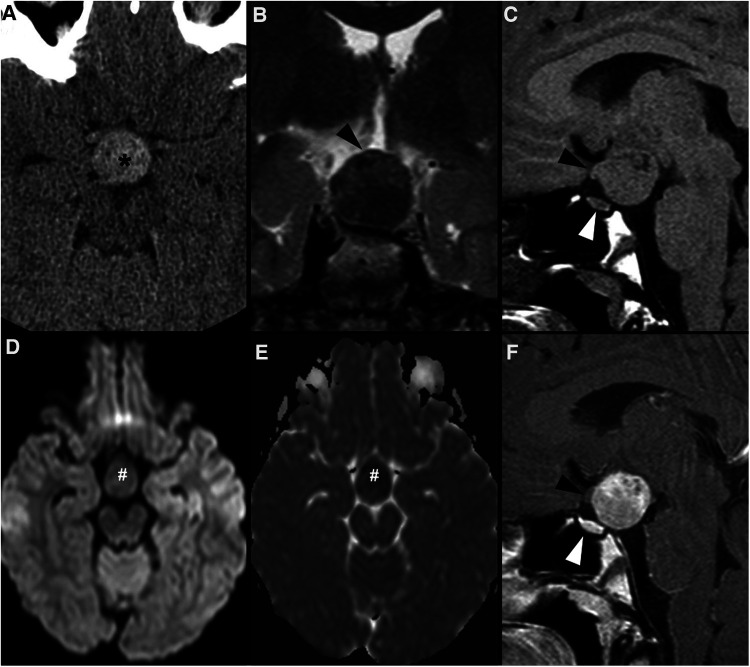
Granular cell tumor of the neurohypophysis in a 12-year-old boy with visual disturbances and central diabetes insipidus. Imaging shows a compact, solid and rounded suprasellar lesion, spontaneously hyperdense (asterisks) on axial head CT scan (A). Brain MRI shows that the mass is well-defined and hypointense on coronal T2WI (B) and isointense on sagittal T1WI (C) (black arrowheads). There is mild reduced diffusivity on axial DWI (D) and ADC map (E), and homogenous solid enhancement on post-gadolinium sagittal T1WI (F). The expansile lesion causes superior and anterior displacement of the optic chiasm (black arrowheads in C,F) and deformation of the floor of the third ventricle. Note normal location and enhancement of the pituitary gland on sagittal images [(C,F) white arrowhead].
